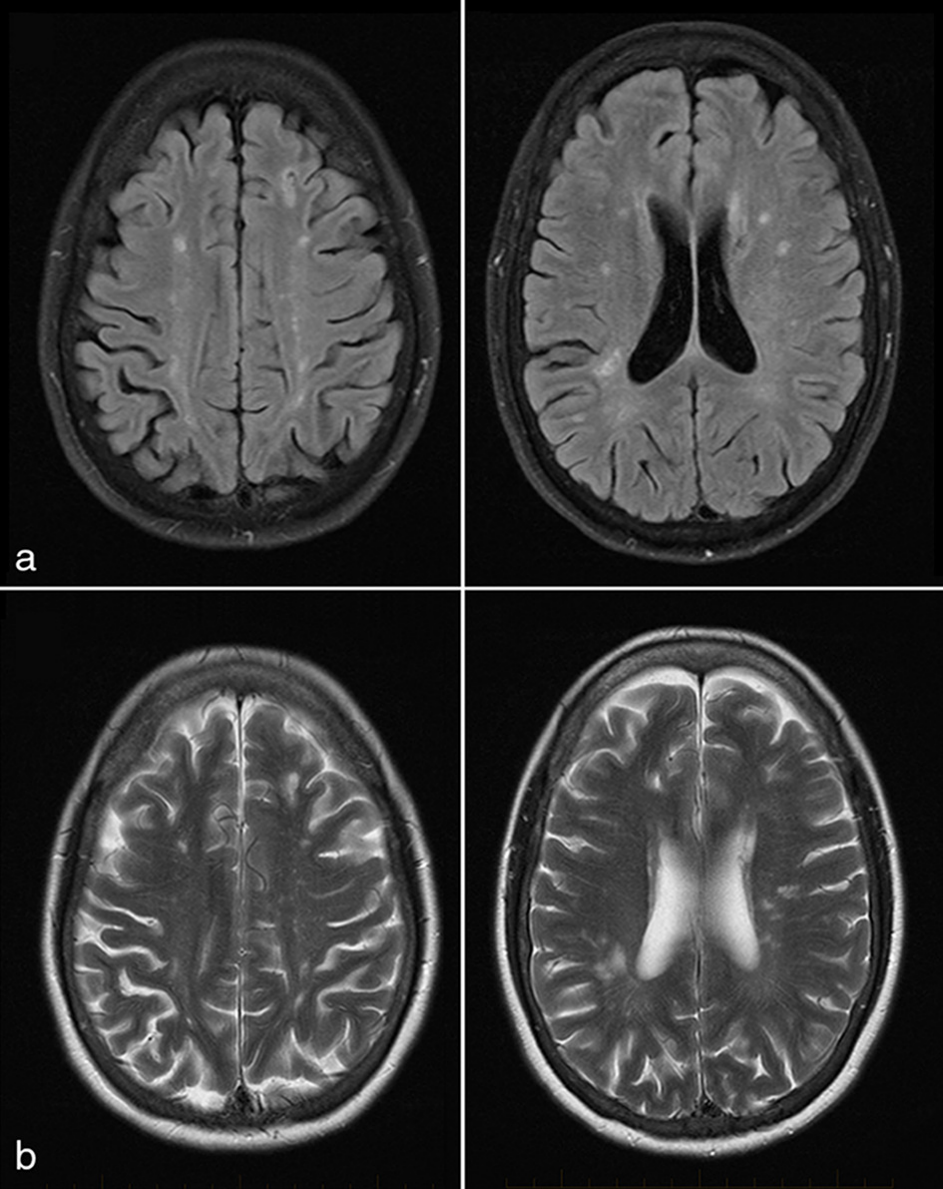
(a) Fluid-attenuated inversion-recovery and (b) *T*_2_ weighted images. A follow-up MRI performed 12 months after completion of rituximab therapy did not show any brain lesions.
